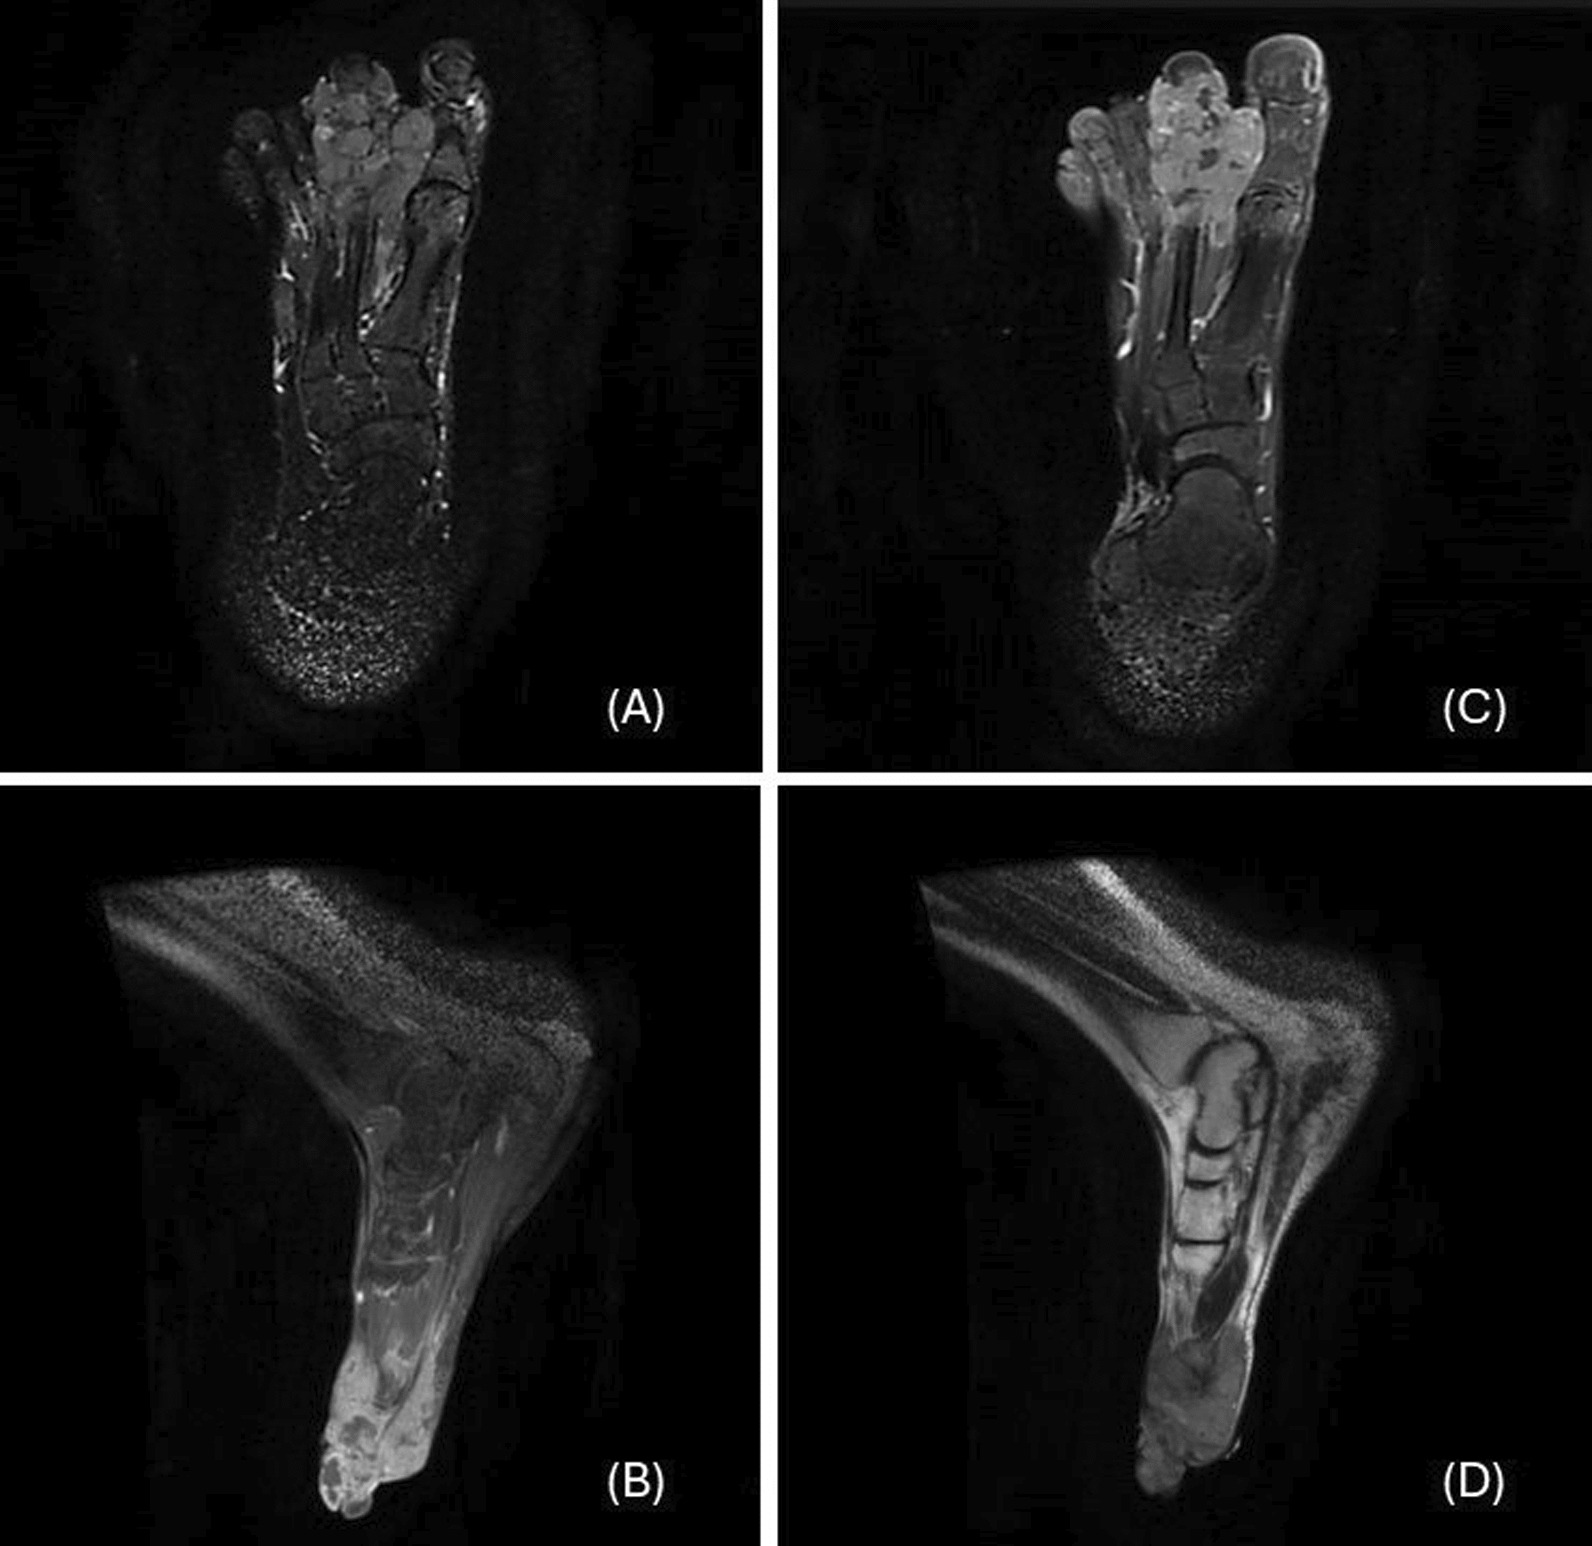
Magnetic resonance imaging assessment of the tumor process. A. T1-weighted axial image shows the tumor without contrast enhancement, providing detailed information about its internal structure. B. T1-weighted sagittal image following the administration of gadolinium contrast, it demonstrates a loss of signal intensity from the majority of the first and second phalanges, along with filling of the first and second interphalangeal joints. C. T2-weighted axial image. D. T2-weighted sagittal image. These finding offers a comprehensive visualization of the tumor’s impact on the surrounding anatomy
